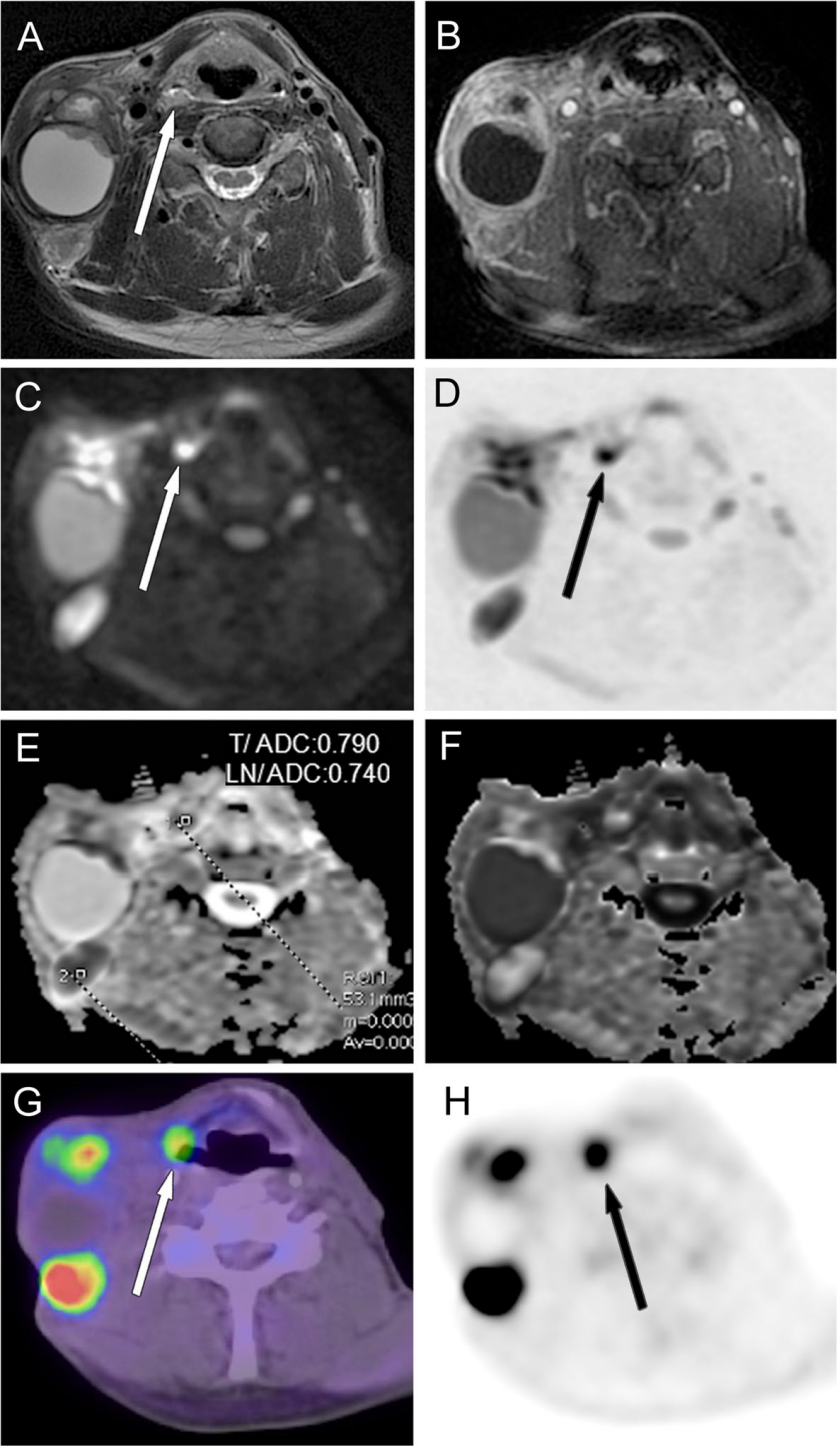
Images of a 56-year-old male with a large right-sided LN metastasis of a small SCC in the right piriform recess. T2W-STIR (a) and CET1WFS (b) show small soft tissue swelling in the entrance of the right piriform recess; ipsilateral kolliquated LNs can be observed. Findings also revealed substantial diffusion restriction with signal increase in axial trace DW images (c and d, *b*-value: 1,000 s/mm^2^) and a corresponding signal decrease in the ADC map (e) and a signal increase in the e-ADC image (f). Note the ADC values, which measured 0.790 × 10^−3^ mm^2^/s in the PT and 0.740 × 10^−3^ mm^2^/s in the LN. On the axial PET-CT fusion image (g) and on the FDG-PET scan (h), increased uptake corresponding to the occult PT (*arrow*) and metastatic nodes can be seen. SCC of hypopharynx, stage T1N3M0
